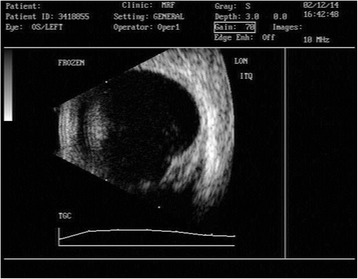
Ultrasound B scan image of the left eye showing indentation by the scleral nodule.
